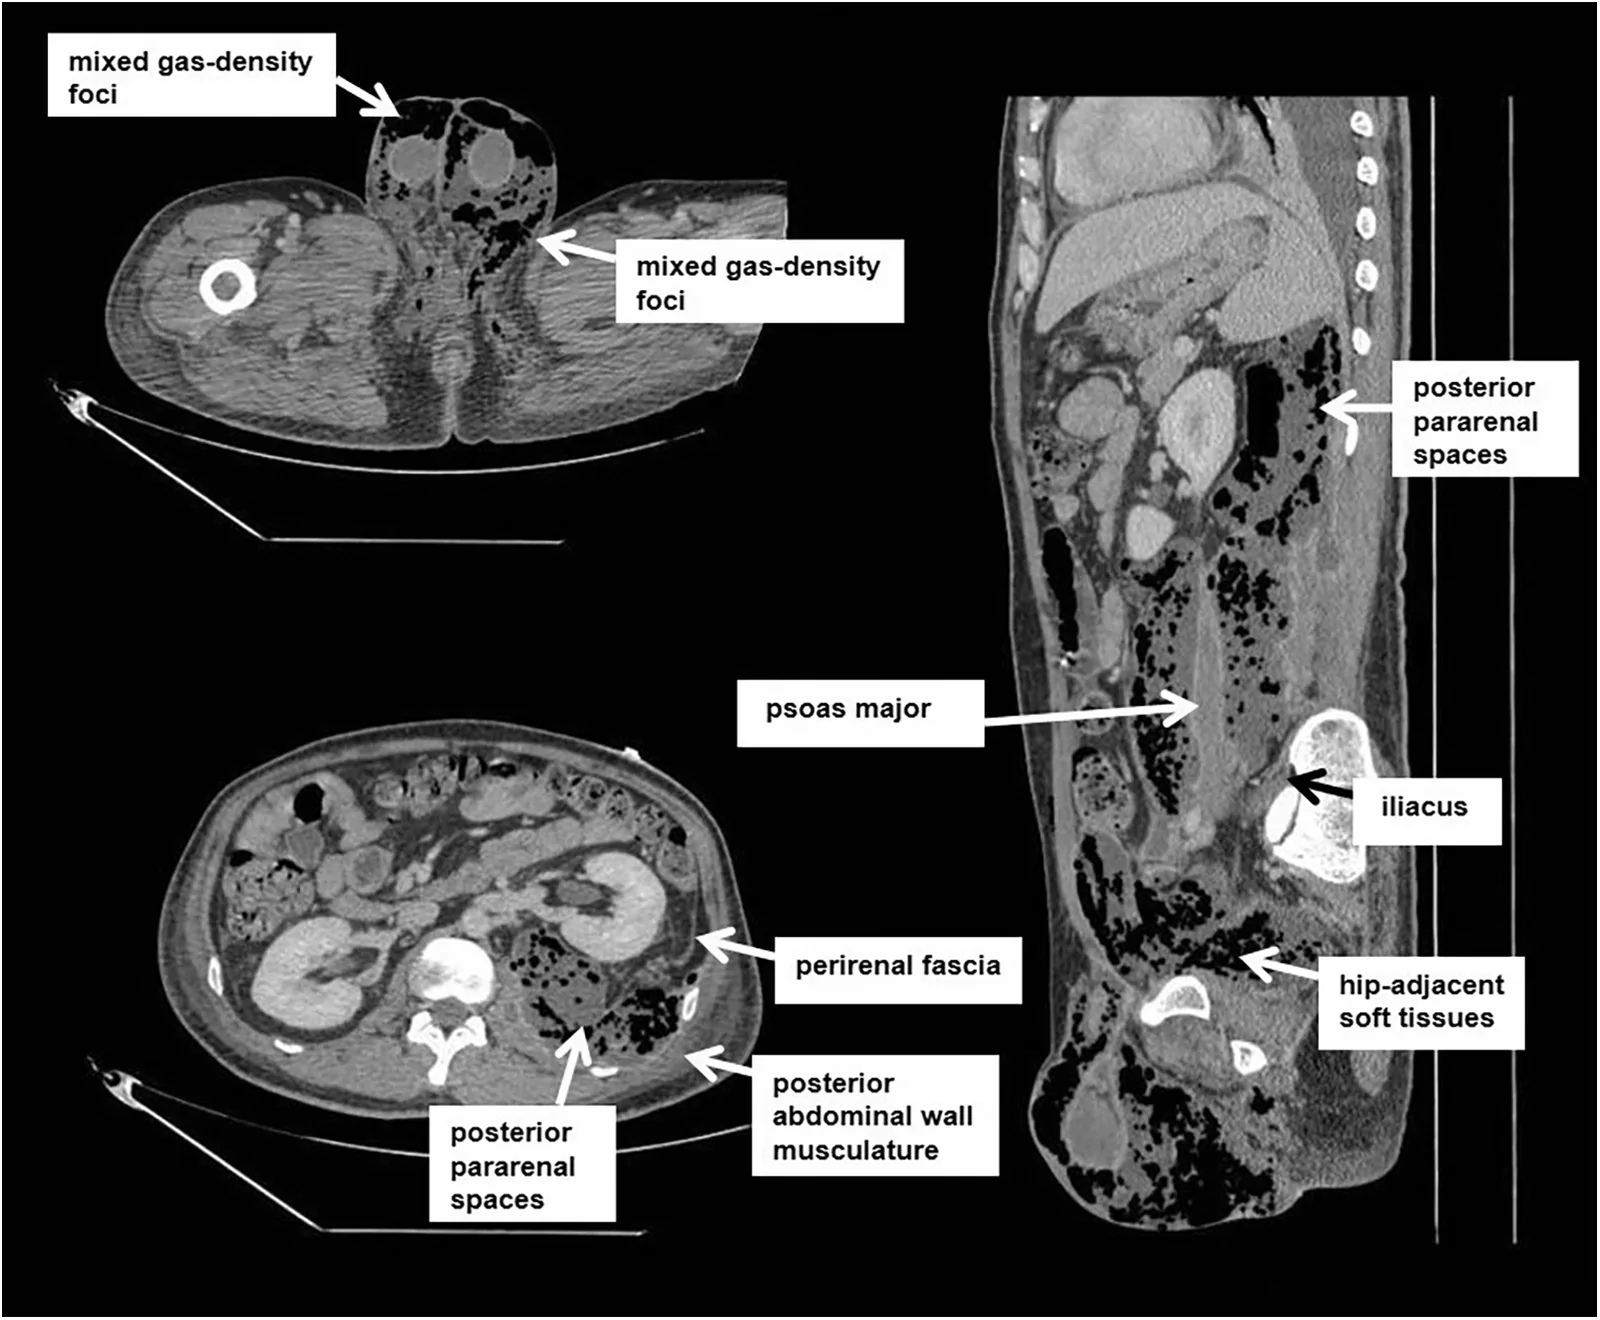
Contrast-enhanced abdominopelvic CT showed bilateral scrotal swelling with mixed gas-density lesions extending superiorly to the pelvis and abdominal wall, with involvement of the left psoas and iliacus muscles, left perirenal fascia, and perirenal spaces, indicating extensive disease.
